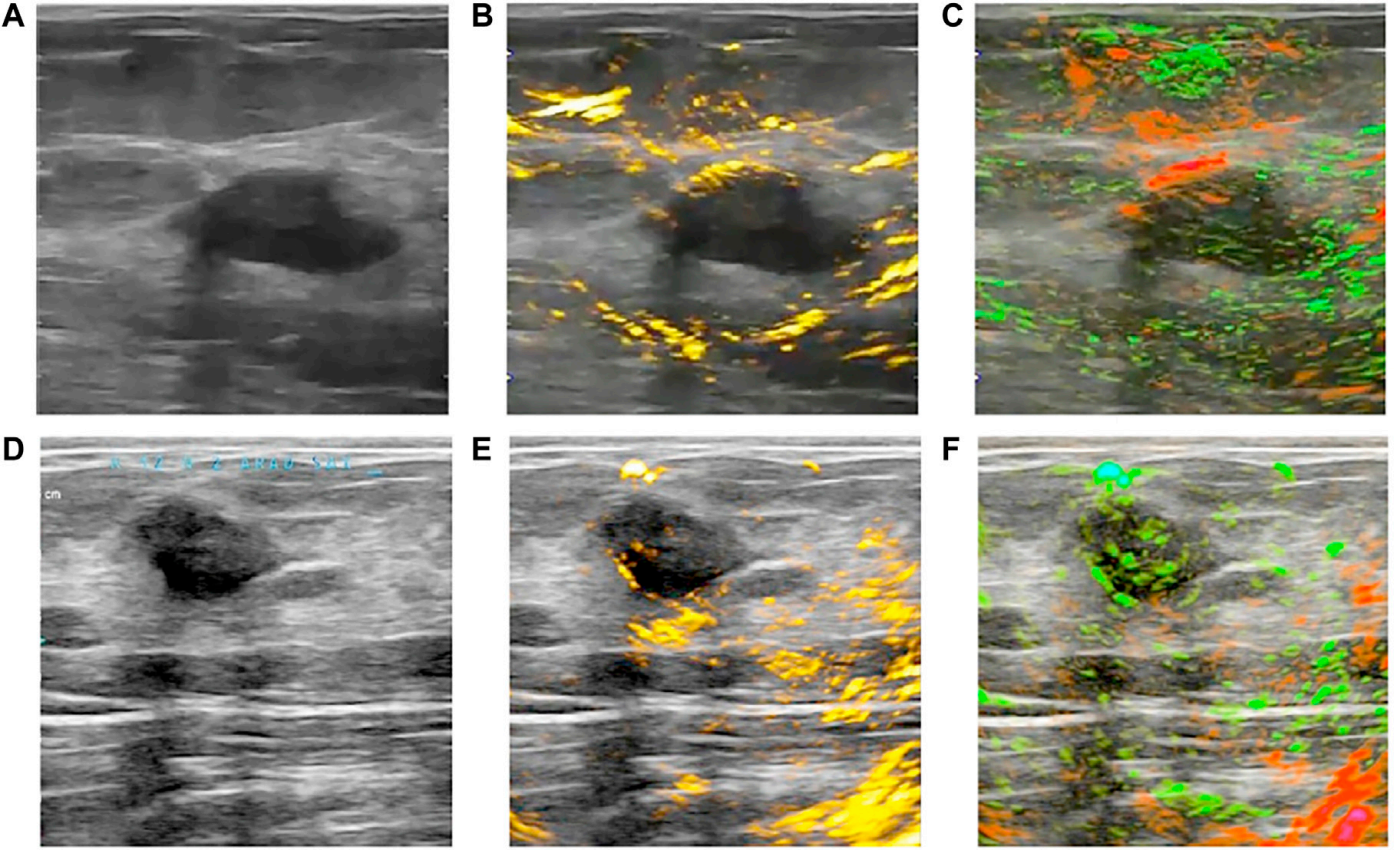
Clinical OA/US imaging of two benign breast tumors. (A,D) show US of two breast tumors; (B) reveals a lack of angiogenesis microvasculature within the breast tumor in OA/US image; (C) shows the majority of the tumor is normally oxygenated in OA/US image; (E, F) display another similar breast tumor in OA/US image. OA: optoacoustic; US: ultrasound. Reprinted with permission from Oraevsky et al. (2018).
